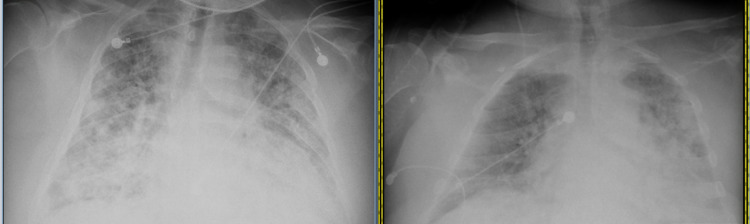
Chest X-ray showing worsening of infiltrates (A) from prior visit study (B)
